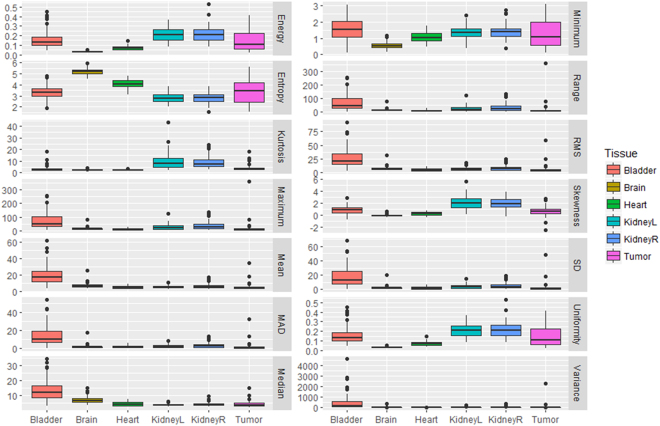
Distribution of 14 SUV-based radiomics features in all segmented volumes. Legend: MAD: Maximum Absolute Deviation, RMS: Root Mean Square, SD: Standard Deviation, KidneyR: Kidney Right, KidneyL: Kidney Left.
